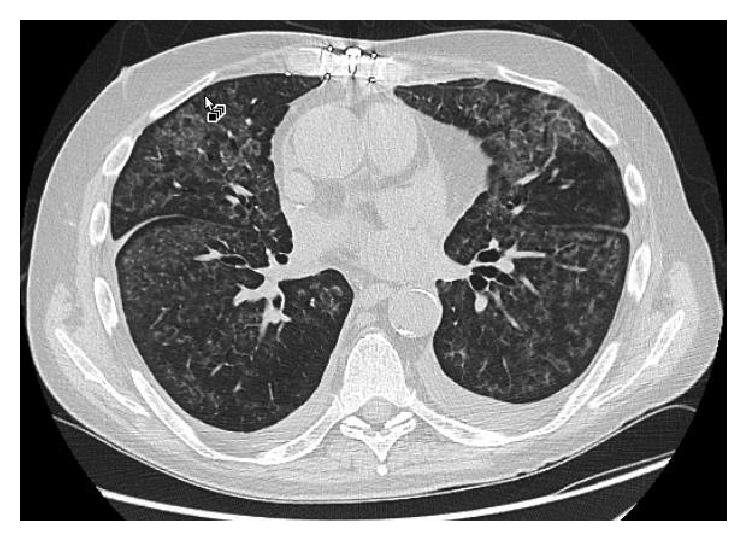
High-resolution axial computed tomography scan of the chest shows diffuse ground-glass abnormalities with interlobular septal thickening in the characteristic “crazy paving” pattern after initiation of everolimus therapy.
